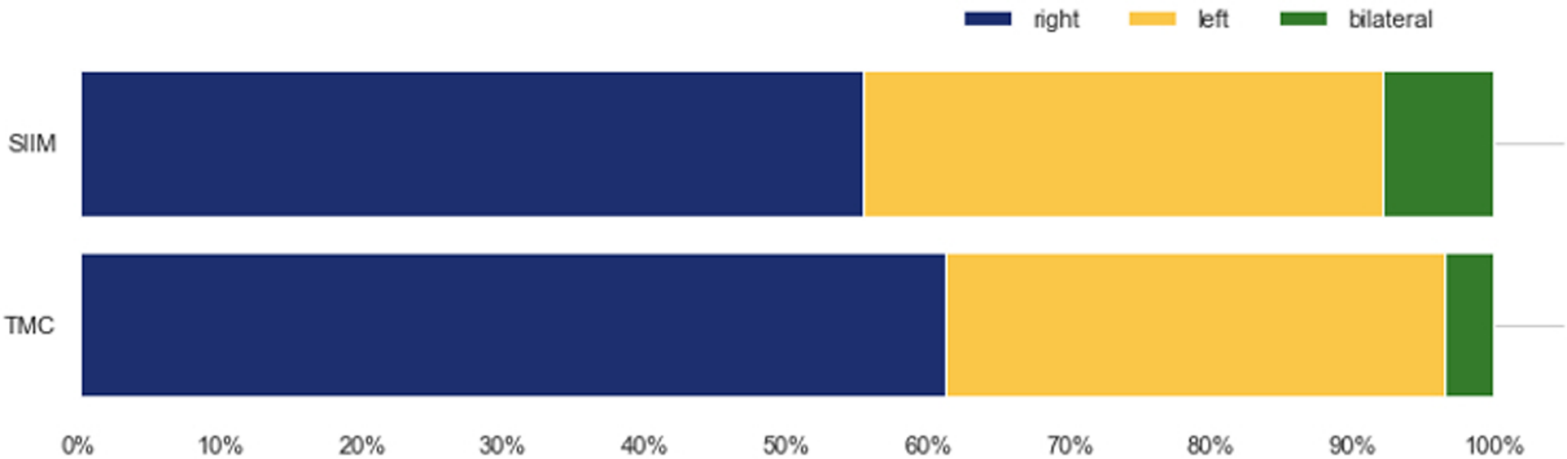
Laterality of pneumothorax on the affected chest X-rays in the SIIM and TMC datasets.
